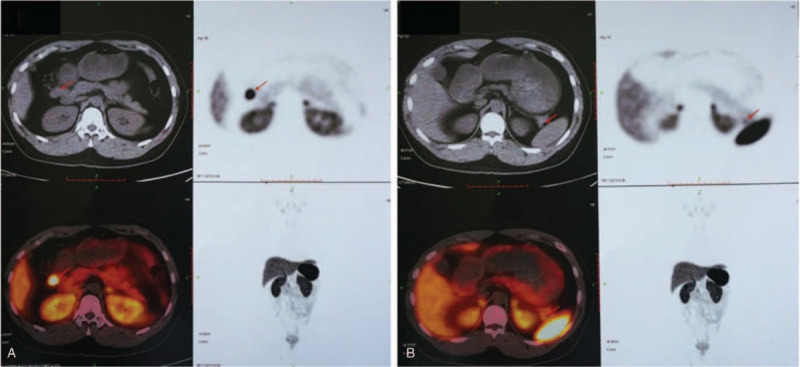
^68^Ga-DOTATATE PET/CT showed increased uptake of tracer in the nodule on the right side of the gastric antrum, no abnormal concentration of tracer was seen in the pancreas (A). The nodule in the tail of the pancreas showed no abnormal concentration of tracer (B).
